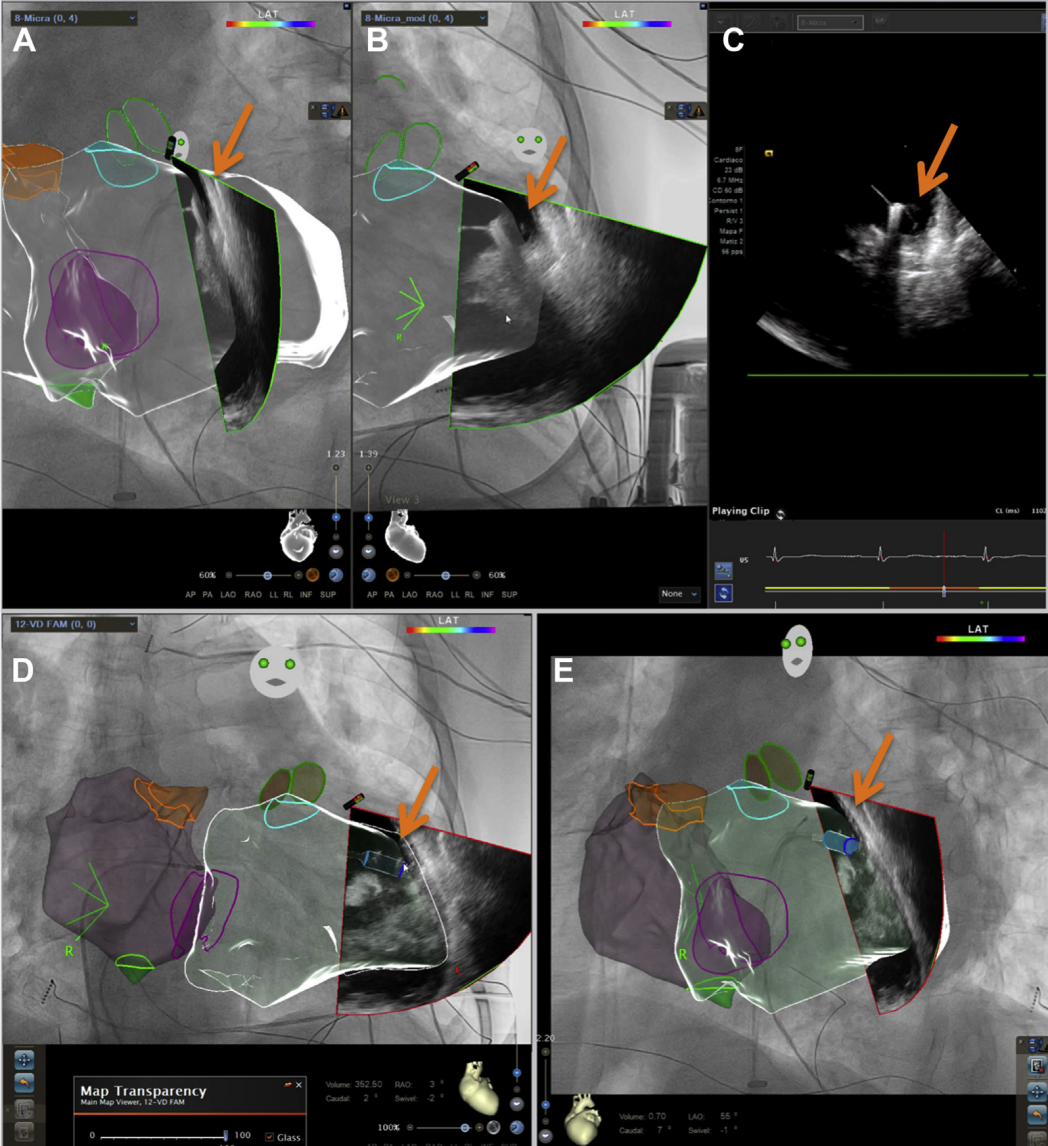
A, B: Intracardiac imaging catheter (ICE) combined with 3-dimensional ultrasound reconstruction of the right ventricle (RV) using the CARTOSOUND (Biosense Webster, Diamond Bar, CA) in right anterior oblique (RAO) and left anterior oblique (LAO) view. C: ICE view only. D, E: Catheter combined with 3-dimensional ultrasound reconstruction of the RV using the CARTOSOUND in RAO and LAO view, with graphic enhancement for clear identification of leadless pacemaker (LPM). (*Arrows* indicate position of LPM.)
